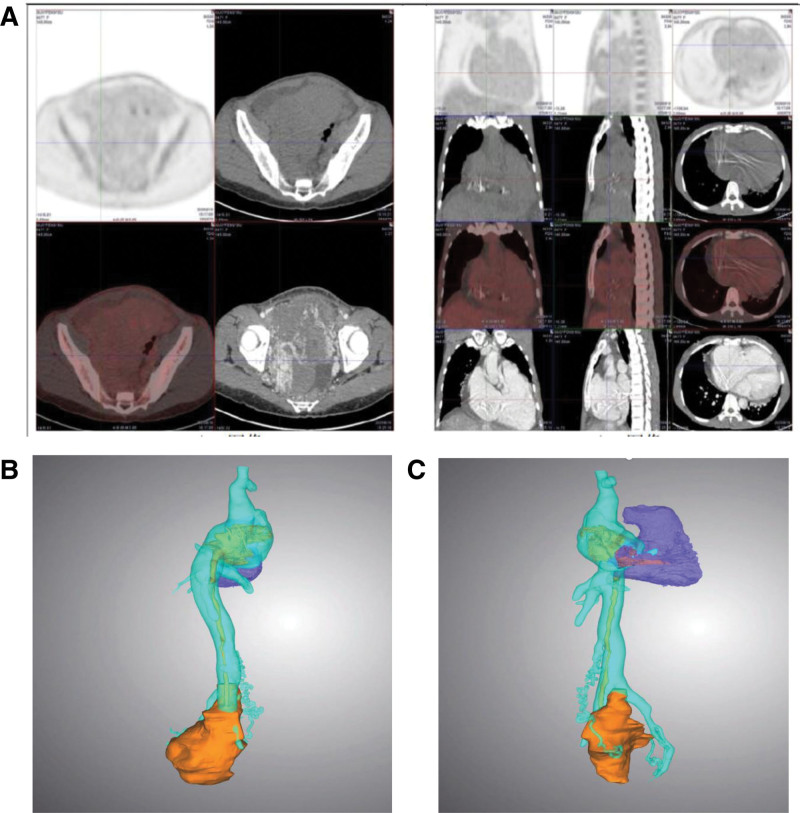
Multiple uterine fibroids: A mass with calcification extending from the right external iliac vein → IVC → right atrium, suggestive of IVL (likely uterine origin). (A–C) No evidence of malignant hypermetabolic lesions elsewhere on whole-body ^18^F-FDG PET/CT. ^18^F-FDG = ^18^F-fluorodeoxyglucose, IVC = inferior vena cava, IVL = intravenous leiomyomatosis, PET/CT = positron emission tomography/computed tomography.
